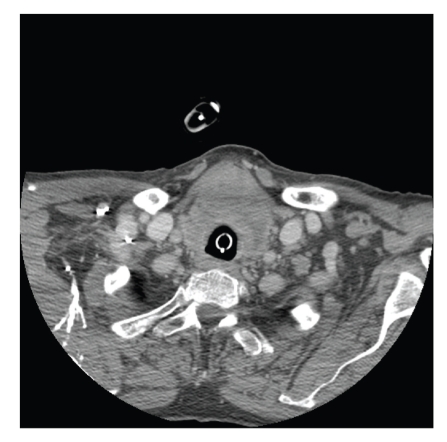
Thyroid mass at the level of the inflated endotracheal tube cuff; the cuff at this level is presumed to be stenting the tracheal airway surrounding the tube. Due to the emergent nature of the case, an extubated radiograph was unable to be obtained.
